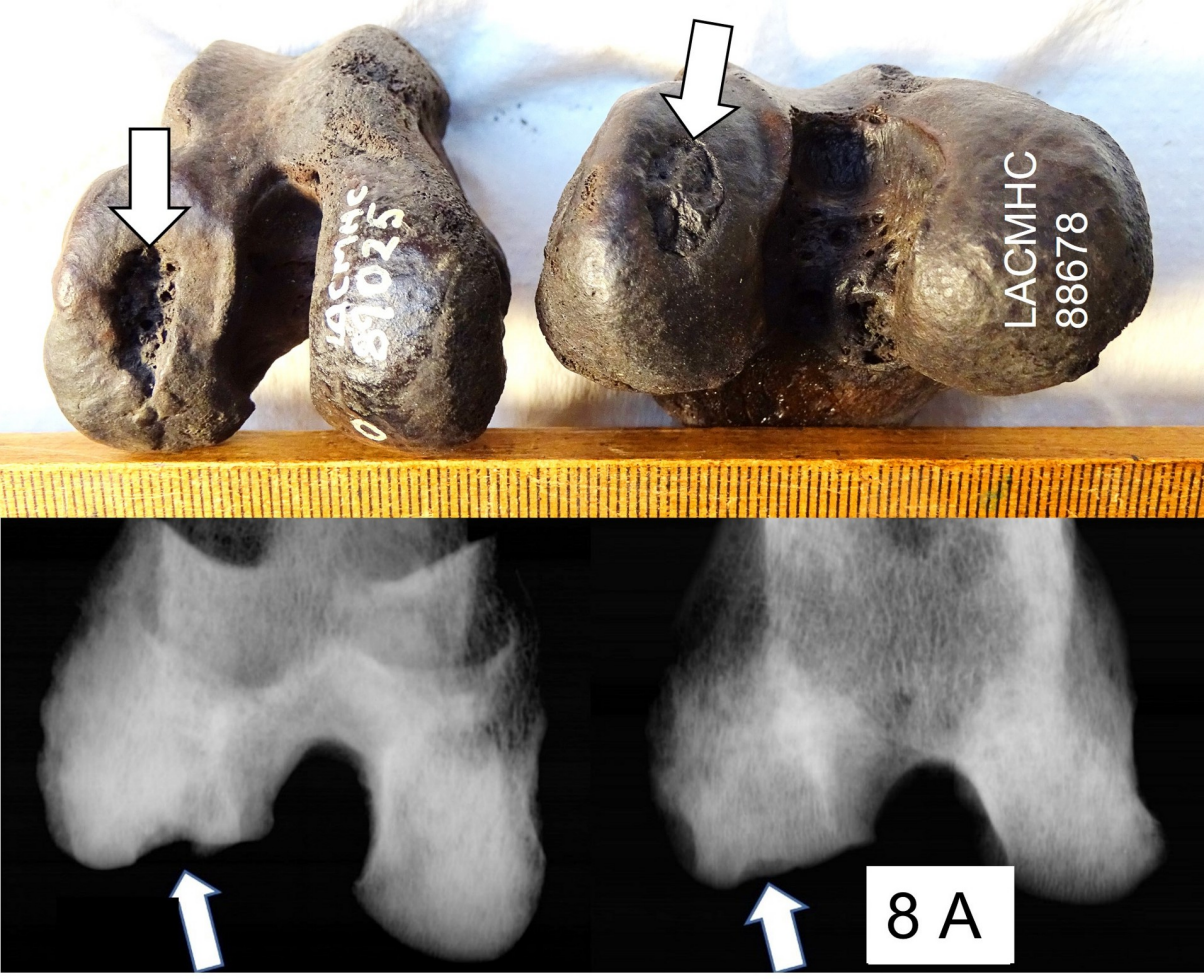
Large subchondral femoral defects in *A*. *dirus L*CMHC 89025 and LACMHC 88678, no secondary osteoarthritis. The defect of LACMHC 89025 is deeper and has sharper edges visible also on the corresponding radiographs.
